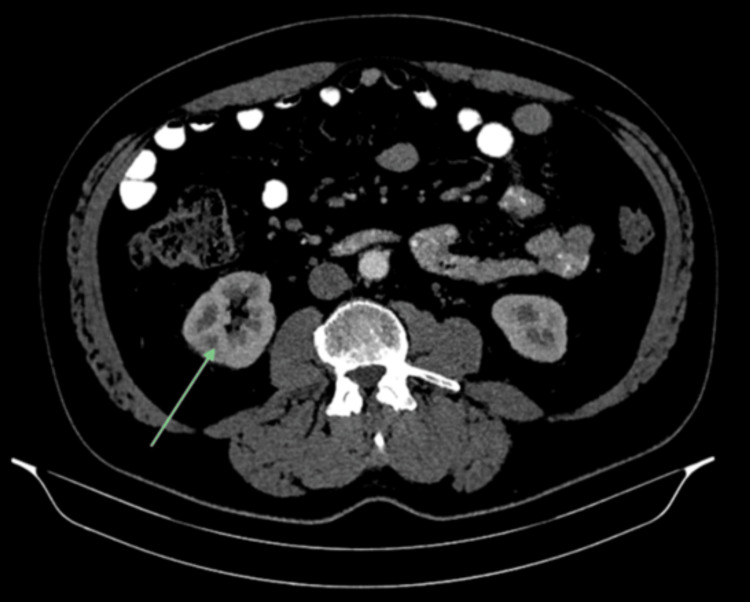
Follow-up CT abdomen, showing resolution of the right-sided focal nephronia with preserved renal parenchymal enhancement
